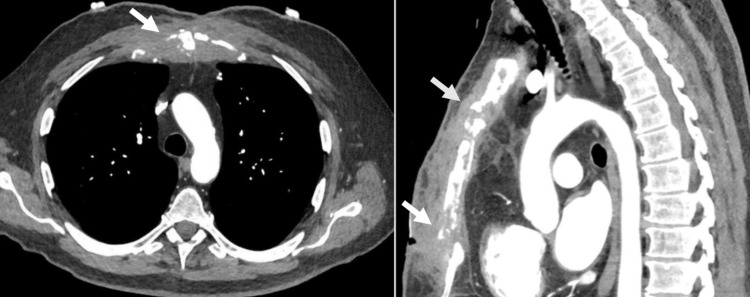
Computed Tomography of Thorax Axial and sagittal computed tomography images of the thorax showing destructive osseous lesions of the sternum (arrow)
